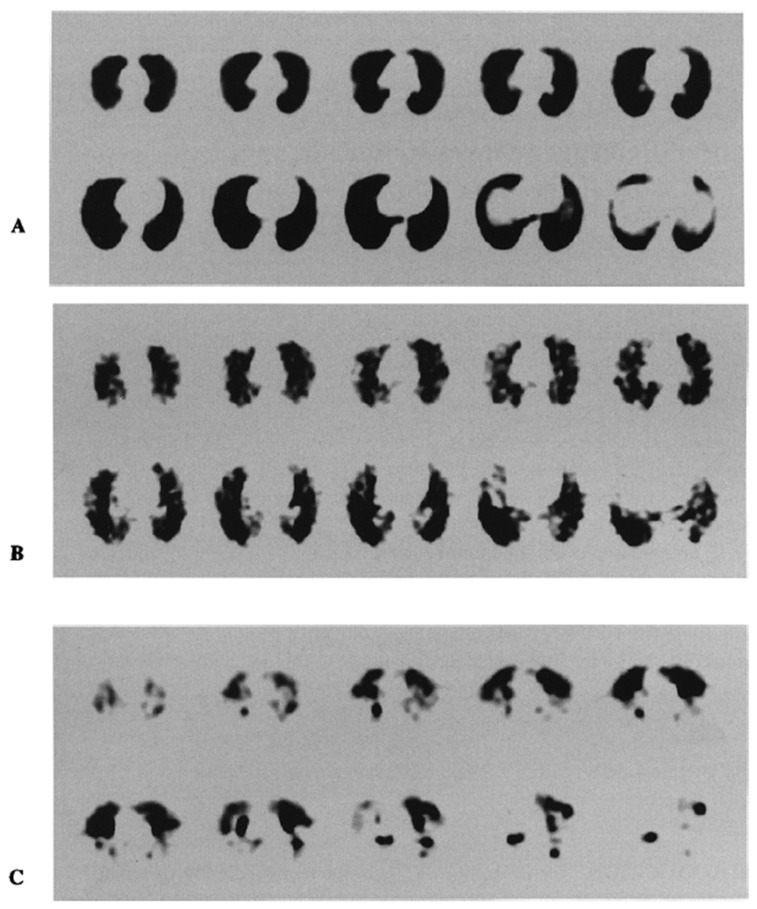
(A) A 45-year-old healthy male. Technegas SPECT images show homogeneous distribution in whole lung. (B) A 65-year-old male with mild emphysema. Technegas SPECT images show heterogeneous distribution in whole lung. (C) A 63-year-old male with severe emphysema. Technegas SPECT images show heterogeneous distribution of cold and hot spots throughout peripheral lung field. Reprinted/adapted with permission from Ref. [36]. 2002, Springer Nature.
